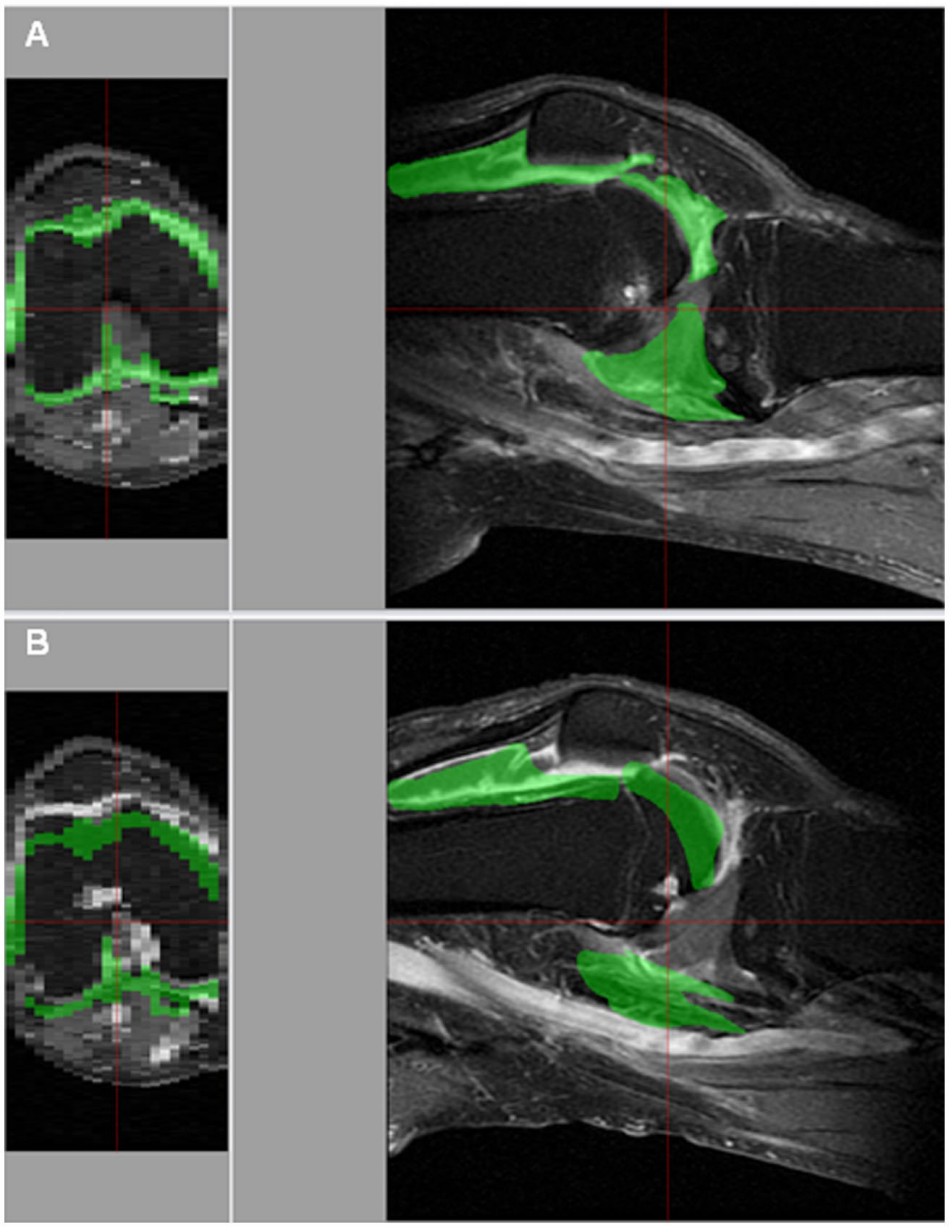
Semi‐automated quantitative assessment of STV using our approach. Software‐computer interface displays a sagittal T_1_‐w postcontrast fat‐suppressed MRI (right) with overlaid synovitis model (green) and a 3D‐rendered axial plane with overlaid synovitis model (left). (A) Correct positioning of synovitis mask to the target image. (B) Displacement in the posterior direction of the synovitis mask to the target image. The displacement of the synovitis‐shape model can also be seen on the 3D rendered axial plane
